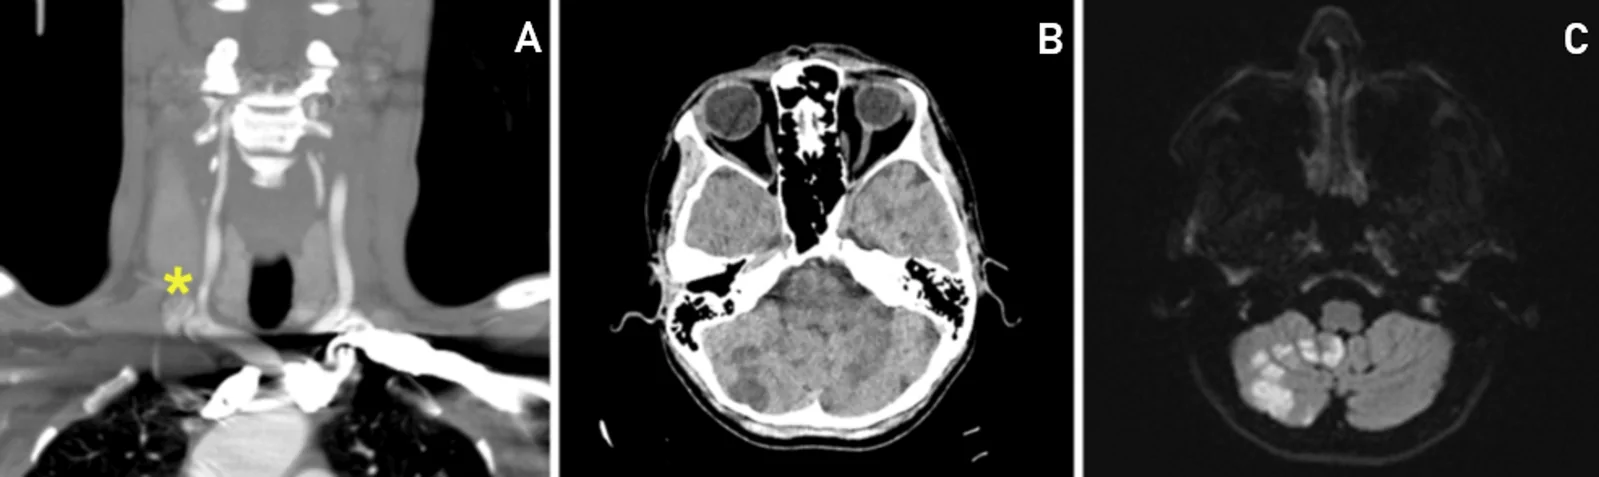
Computed tomography (CT) and magnetic resonance (MR) imaging demonstrate thrombus formation and downstream damage in susceptible vascular territories. (A) CT angiography (CTA) of the head and neck shows thrombus (*) identified within the right subclavian artery just distal to the takeoff of the right vertebral artery. (B) Head CT without intravenous (IV) contrast shows multifocal hypodensities within the right inferior cerebellar hemisphere involving the posterior inferior cerebellar artery distribution with no evidence of hemorrhagic conversion. (C) Brain MR shows multifocal increased diffusion-weighted imaging (DWI) signal with associated mildly decreased apparent diffusion coefficient (ADC) and T2 fluid-attenuated inversion recovery (FLAIR) hyperintensity throughout the right cerebellar hemisphere. The multifocal nature and distribution of infarctions suggest an embolic source.
